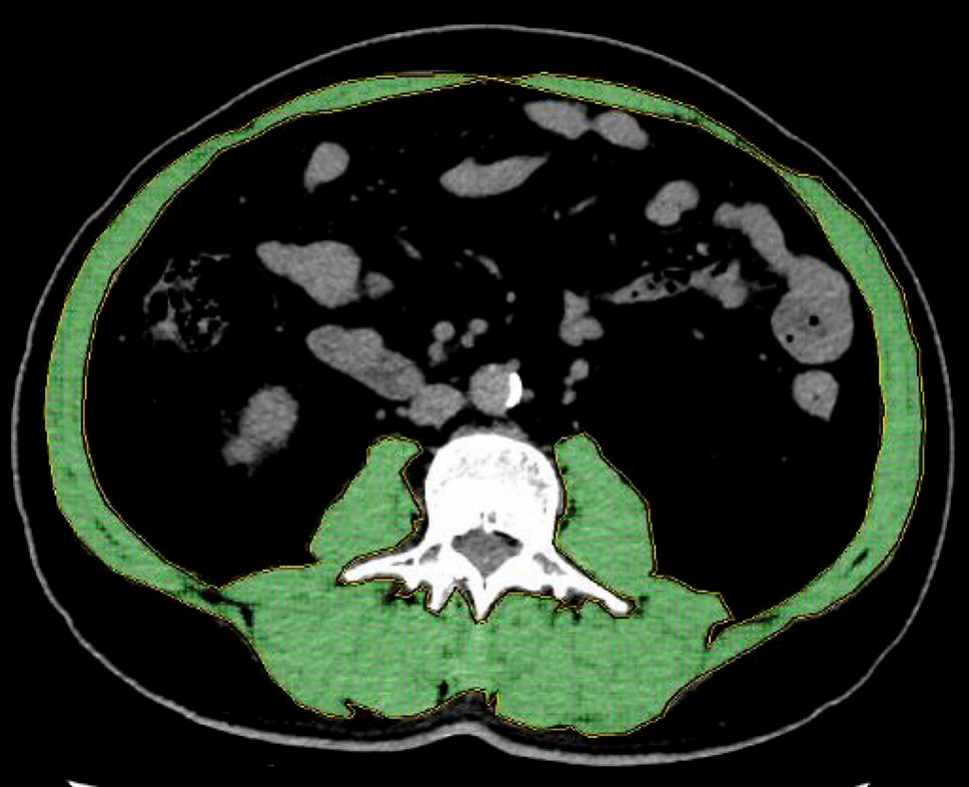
Cross-sectional computed tomography scan at the level of third lumbar vertebra, in which muscle was delineated. The skeletal muscle area (SMA) and mean skeletal muscle density (SMD) can be calculated automatically
